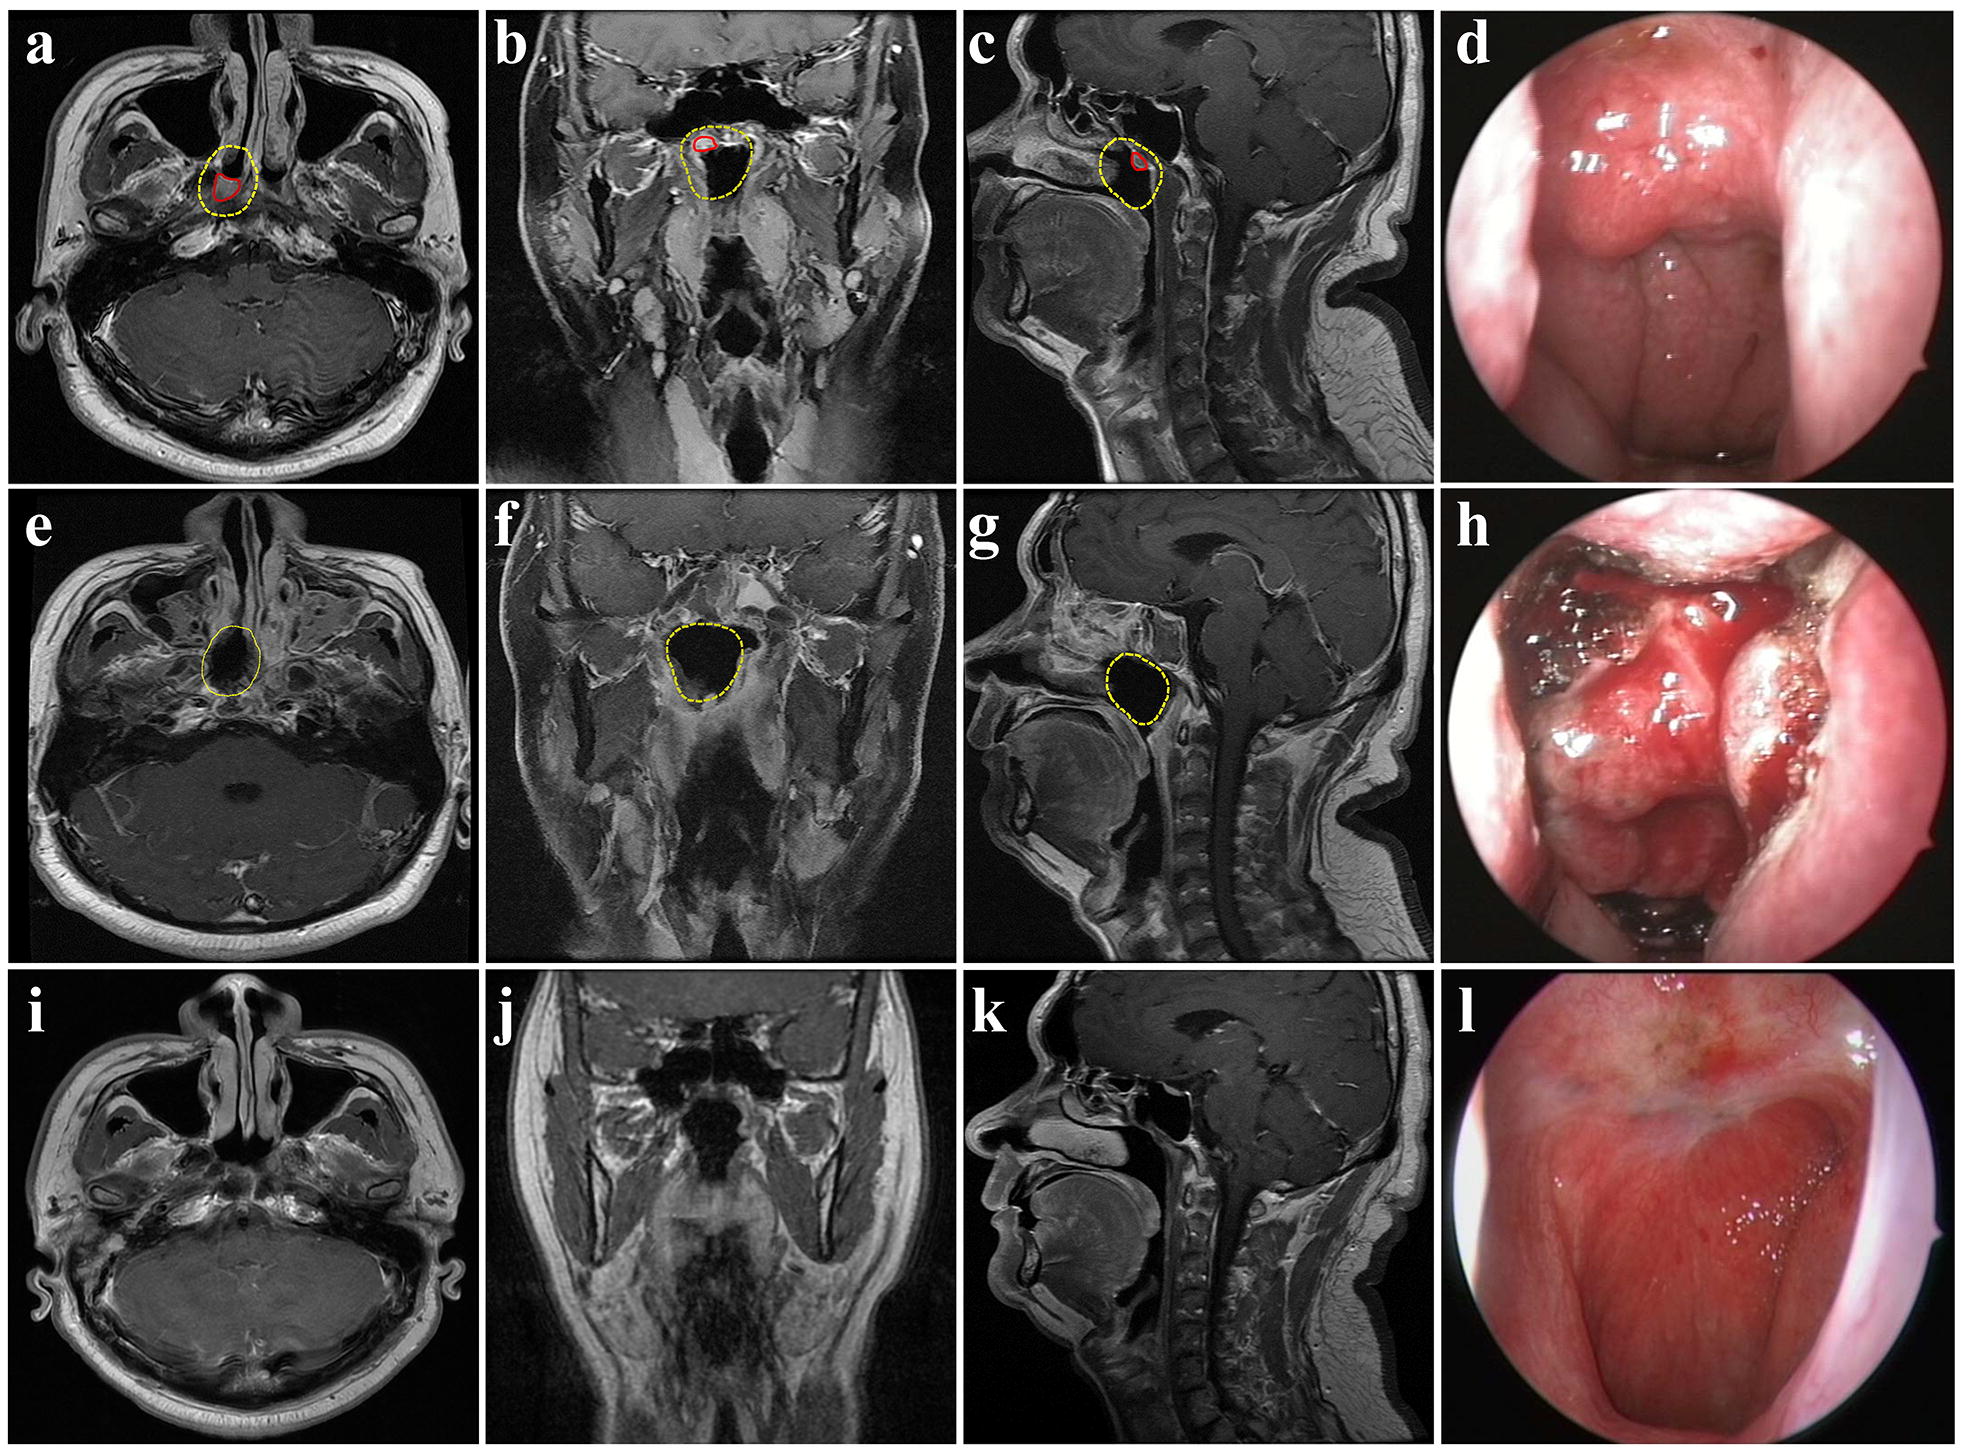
Magenetic resonance and endoscopic images contrast before and after surgery. a–c shown the tumor invasion regions (red line) and surgical margin (yellow line) in preoperative T1-weighted MR images in horizontal, coronal and sagittal view, respectively. The tumor invasion regions were located in the right superior wall of the nasopharynx and the planed surgical margin which was made by our NPC experts team before surgery covered most of the superior wall and part of the posterior wall of the nasopharynx. d was the preoperative endoscopic nasopharyngeal image and shown that nodular masses were found in the right superior wall of the nasopharynx, not involving the bilateral pharyngeal recesses and eustachian tubes. e–g shown the resection regions in T1-weighted MR images in horizontal, coronal and sagittal view, respectively, at 3 days after surgery. The surgical defect was highly similar to the planned surgical margin (yellow line). h shown the endoscopic nasopharynx images during operation, which was marking the resection boundary according to the planed surgical margin delinerated before surgery. i–k shown no abnormal neoplasm was observed in nasopharynx in T1-weighted MR images in horizontal, coronal and sagittal view, respectively, at 10 years after surgery. l shown the synchronous endoscopic nasopharyngeal image and shown the nasopharyngeal defect was re-epithelized and no abnormal neoplasm was observed
